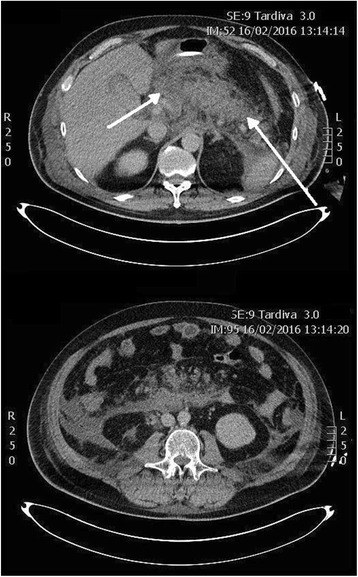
First CT scans at admission. Acute pancreatic necrosis and edema of pancreatic tail and surrounding adipose tissue are visible (*arrows*)
